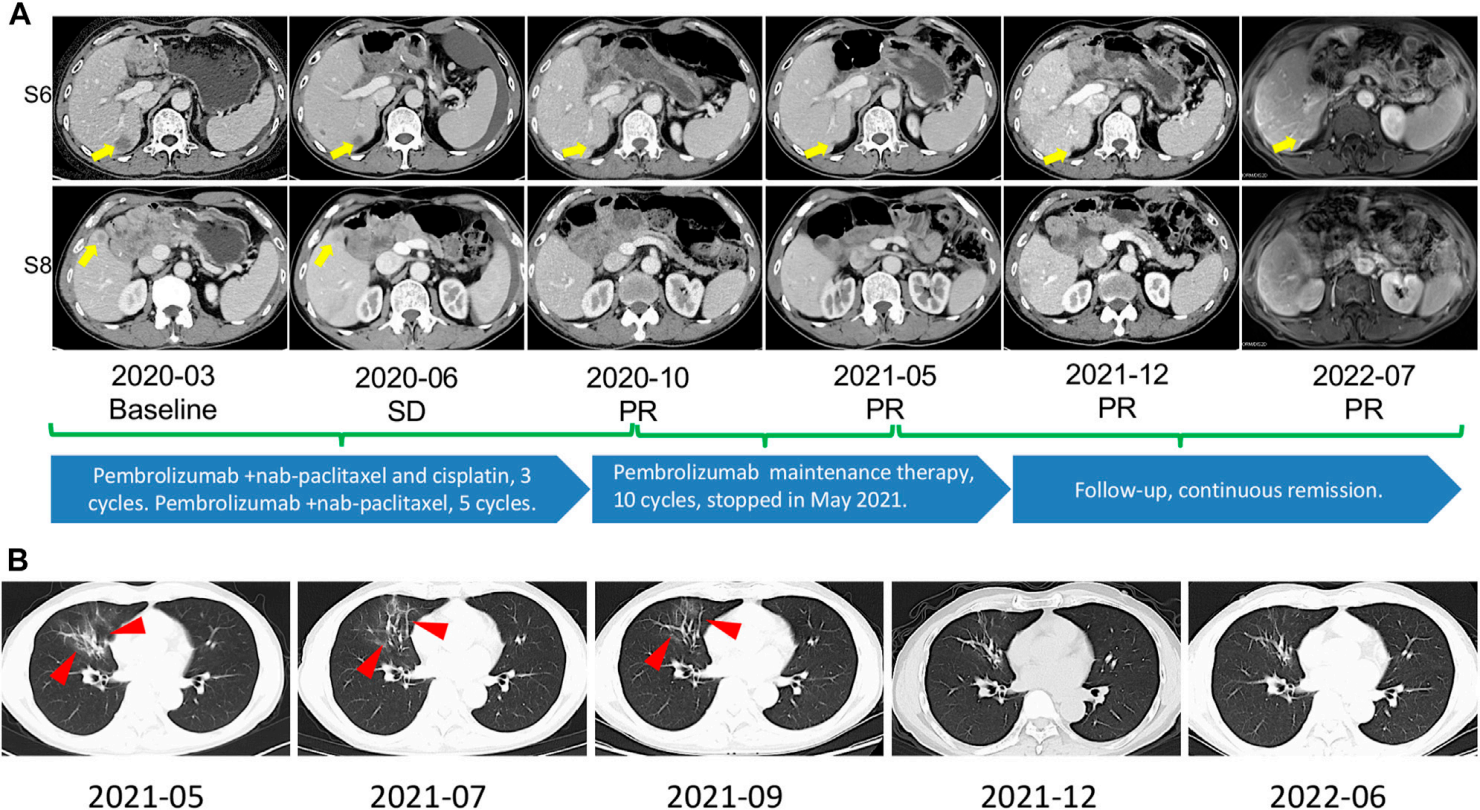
(A) The timeline and representative CT and MRI images. CT of March 2020, yellow arrow points toward intrahepatic lesions in S6/S8 at tumor metastasis. CT of June 2020 revealed stable disease was observed after 2 cycles of nab-paclitaxel/cisplatin plus pembrolizumab. CT of October 2020, partial remission was observed after 3 cycles of nab-paclitaxel/cisplatin plus pembrolizumab and 5 cycles of nab-paclitaxel plus pembrolizumab. CT of May 2021, continuous partial remission was observed after 10 cycles of pembrolizumab maintenance therapy. In July 2022, MRI showed durable partial remission. S6, Segment 6; S8, Segment 8; SD, stable disease; PR, partial remission. (B) Image changes during prednisolone treatment after the onset of CIP. CIP was significantly relieved, as seen in the images. The red arrow points to the part where CIP occurred. CIP, checkpoint inhibitor pneumonitis.
